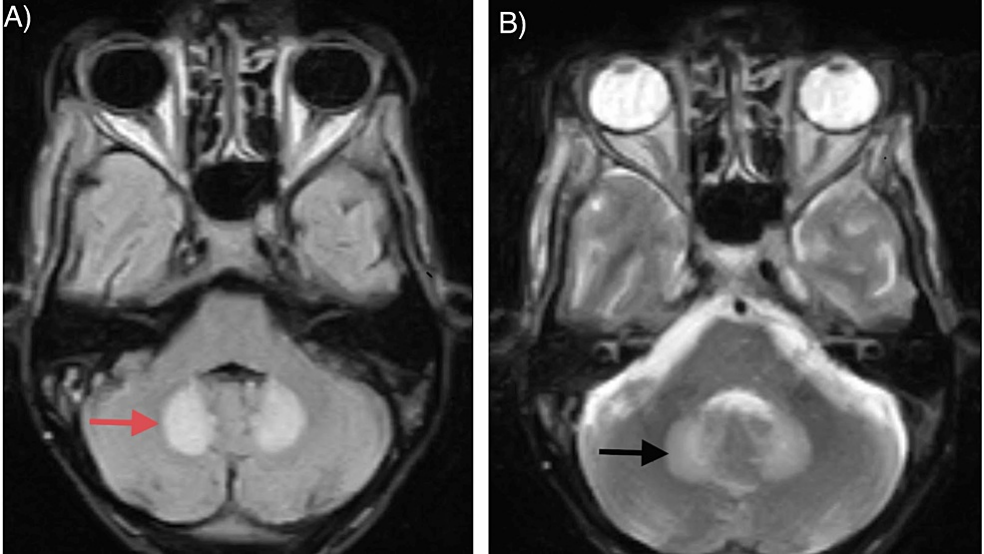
1.5 Tesla MRI of a 58-year-old male with a history of metronidazole intake for pus collection in the abdomen. The (A) axial section of the FLAIR sequence (red arrow) and (B) T2WI (black arrow) demonstrate the characteristics of bilateral symmetric hyperintense lesions in the dentate nuclei of bilateral cerebellar hemispheres. MRI: magnetic resonance imaging; FLAIR: fluid-attenuated inversion recovery; T2WI: T2-weighted image
